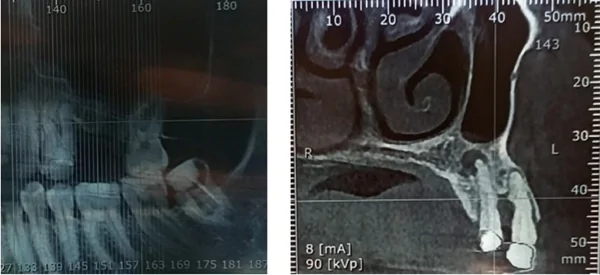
Radiographic examination of the initial situation.
